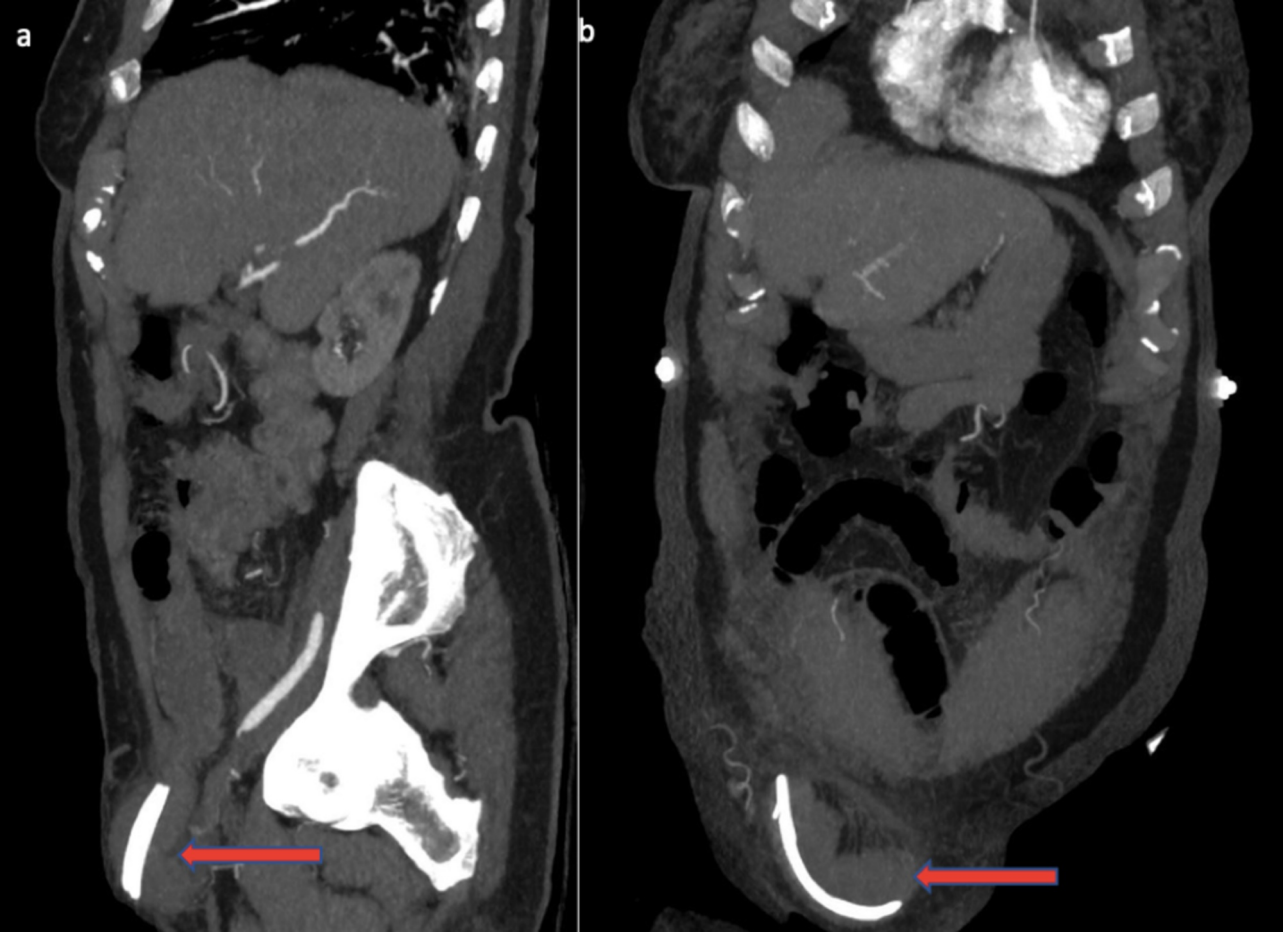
Abdominal CT sagittal (a) and coronal (b) views demonstrating a pair of biliary stents (red arrows) within a bowel containing right inguinal hernia.
